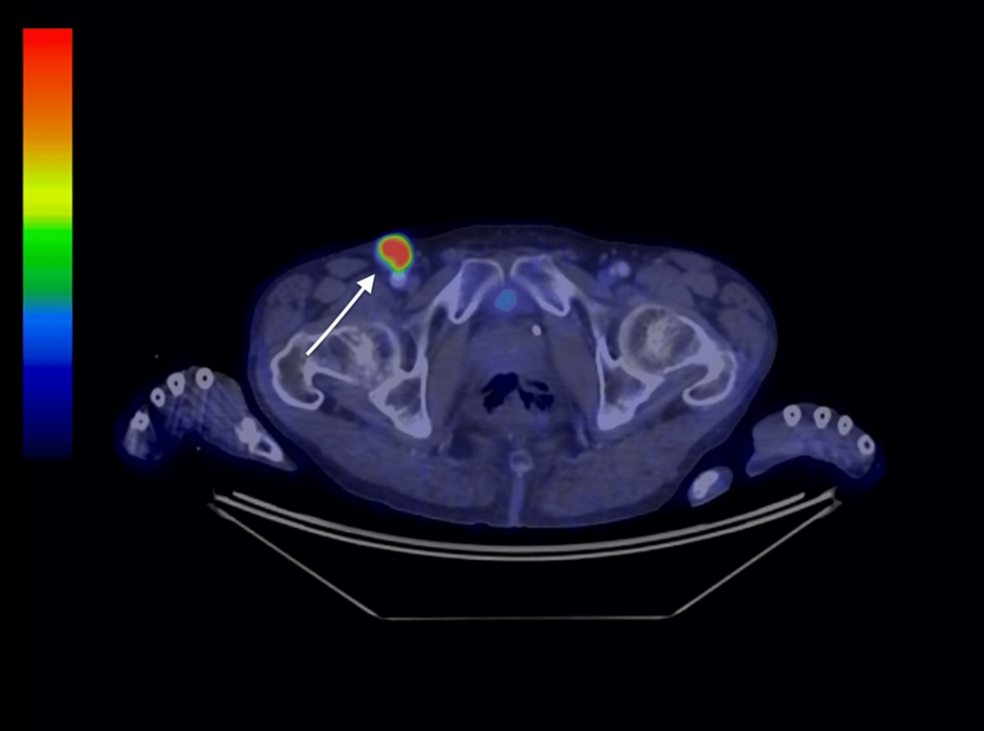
PET CT showing a right inguinal lymph node indicated by a white arrow
